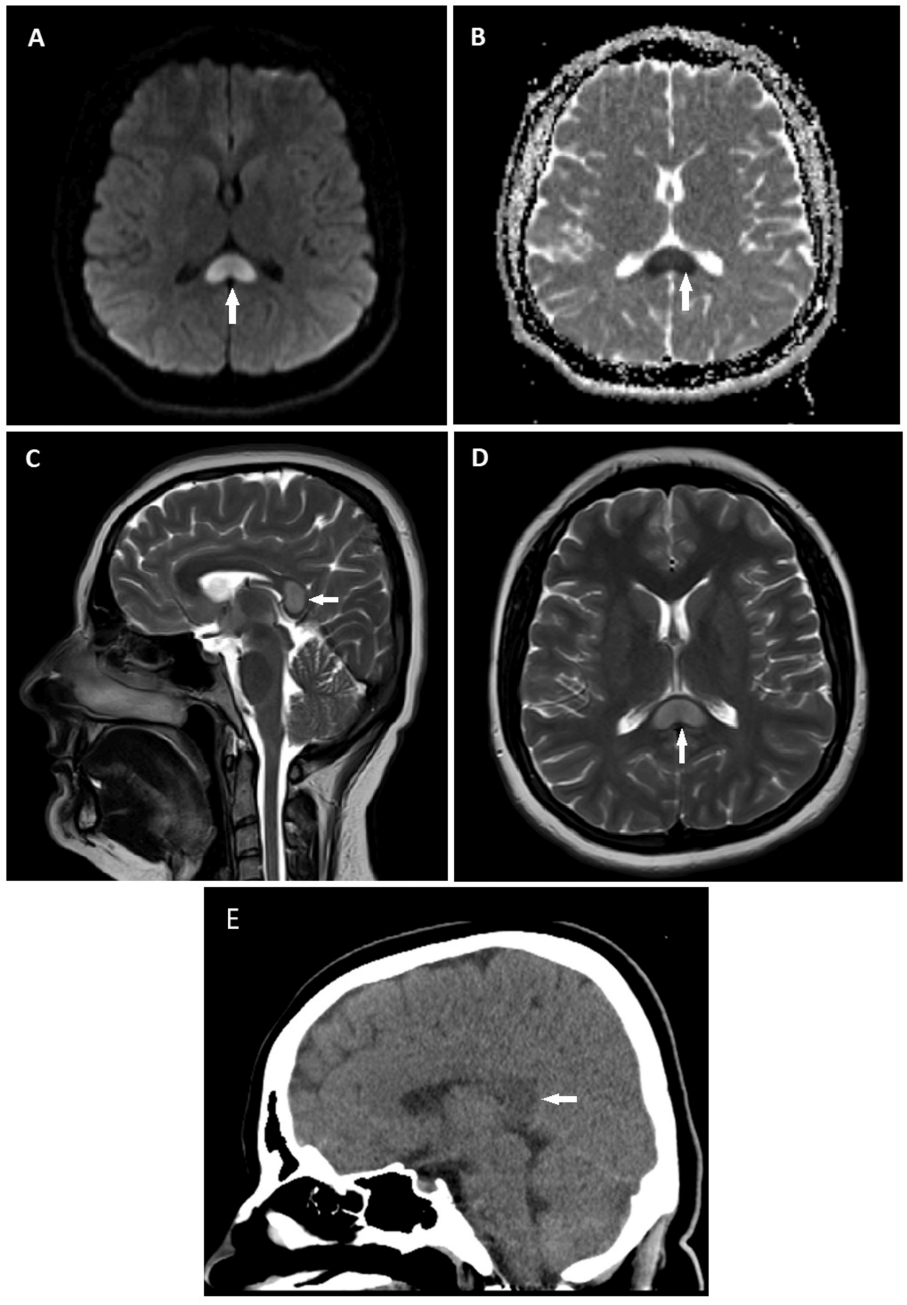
Brain MRI and CT of the case on the day of admission. MRI of the brain shows restricted diffusion in the splenium of the CC with a hyperintense signal on the DWI (A) and a hypointense signal on the apparent diffusion coefficient map (B) (white arrows). The sagittal (C) and axial (D) T2-weighted images reveal the ovoid, well-circumscribed hyperintense lesion within the mid-splenium (white arrows). The sagittal CT image shows a hypodense lesion within the splenium (E) (white arrow). MRI: magnetic resonance imaging; DWI: diffusion-weighted imaging; CT: computed tomography; CC: corpus callosum
